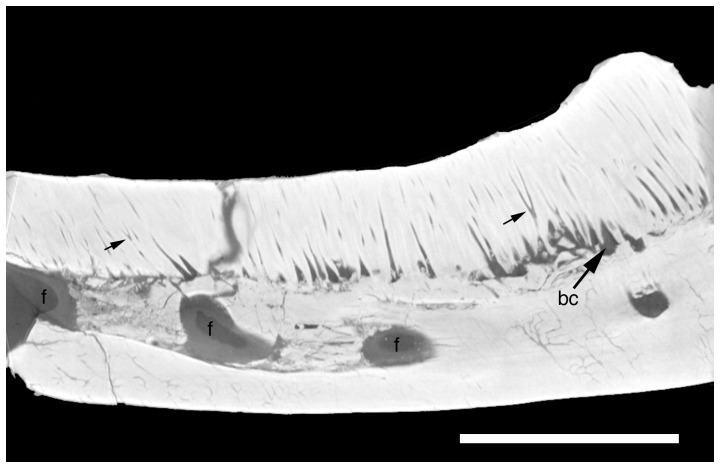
Parasagittal micro CT section of the right dentary of *Oenosaurus muelheimensis*. Posterior is to the right. Abbreviations: bc, basal cavity; f, foramen. Small arrows point to bifurcating dentine channels. Scale bar is 5 mm.
